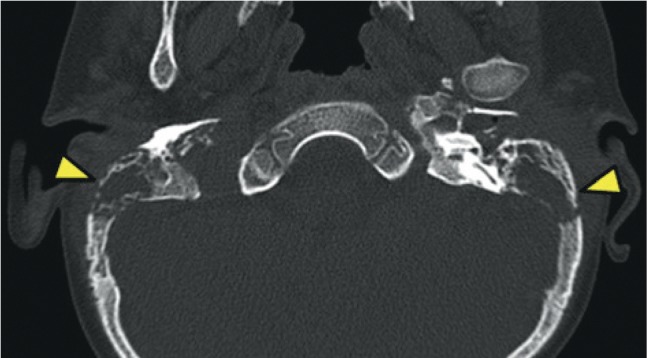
Cortical erosion of the temporal bone (yellow arrows) in a CT scan. Axial view.
